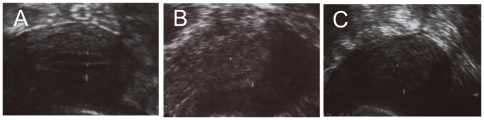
The ultrasonic image of Pattern A, C and SC endometrium.
